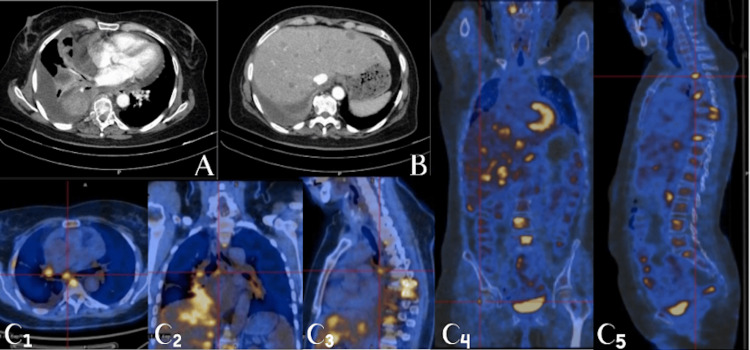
Baseline imaging at diagnosis (A) Contrast-enhanced chest CT demonstrating the primary lung adenocarcinoma in the right lung, associated with bronchial wall thickening and narrowing of the right lower lobe bronchus, resulting in obstructive atelectasis of the right middle and lower lobes. (B) Contrast-enhanced abdominal CT showing multiple hypodense hepatic lesions consistent with hepatic metastatic disease. (C1-C5) Whole-body FDG PET-CT images, including axial, coronal, and sagittal maximum intensity projection views, demonstrating hypermetabolic activity of the primary lung lesion and widespread metabolically active metastatic disease, including extensive osseous involvement, as well as mild FDG uptake along focal pleural thickening adjacent to a small-volume pleural effusion, consistent with Stage IVB disease.
